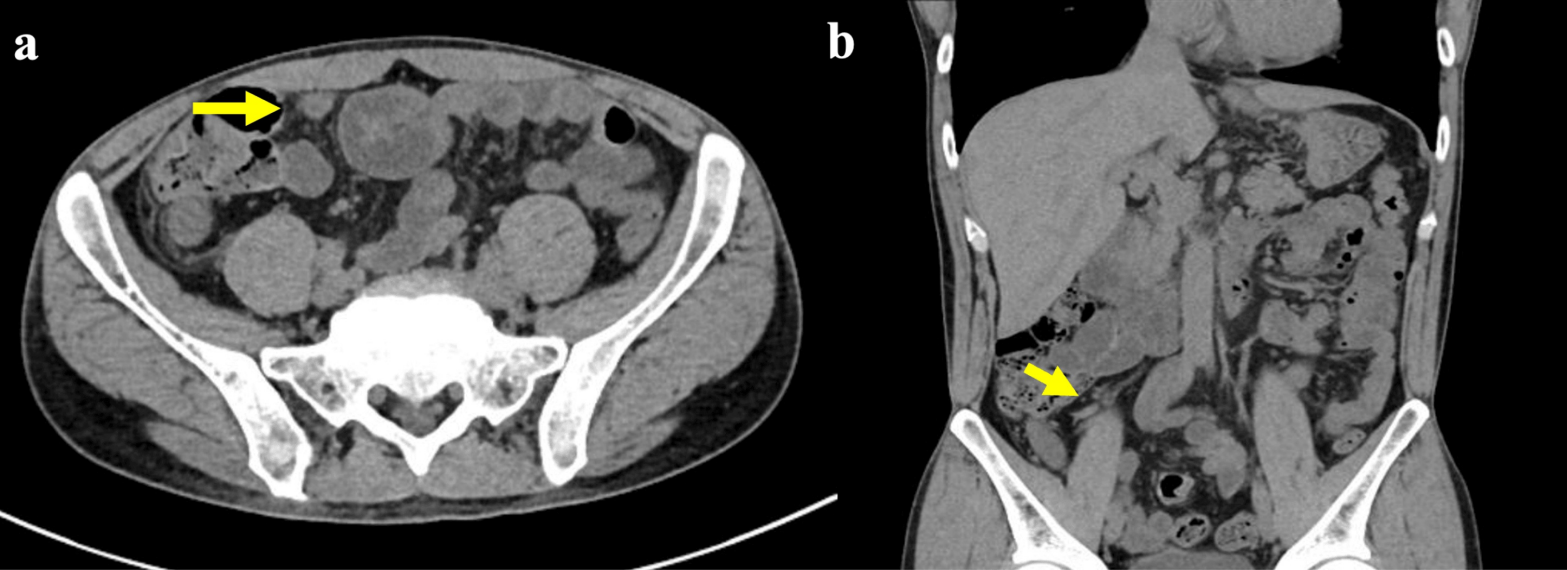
Abdominal computed tomography showing mild enlargement of the appendix. (a) Axial view (b) Coronal view.
